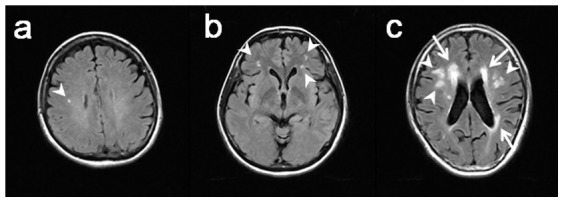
Fluid-attenuated inversion recovery (FLAIR) images of leukoaraiosis. FLAIR images of single subcortical leukoaraiosis (SLA) (a), multiple SLA (b), large multiple SLA (c), and periventricular leukoaraiosis (PLA) (c). Arrow heads and arrows show SLA and PLA, respectively.
